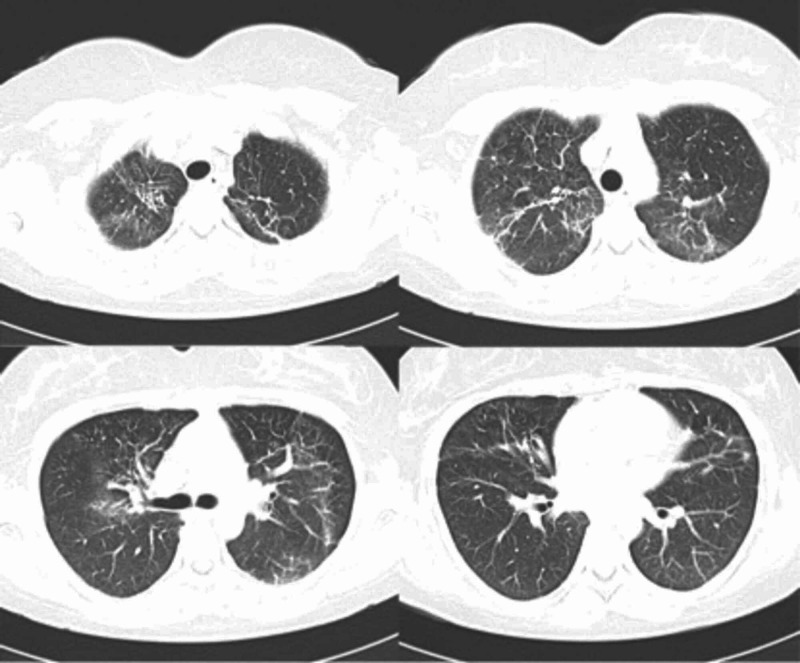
Thorax CT images of the patient after corticosteroid treatment showing resolution of nearly all ground-glass opacities.
